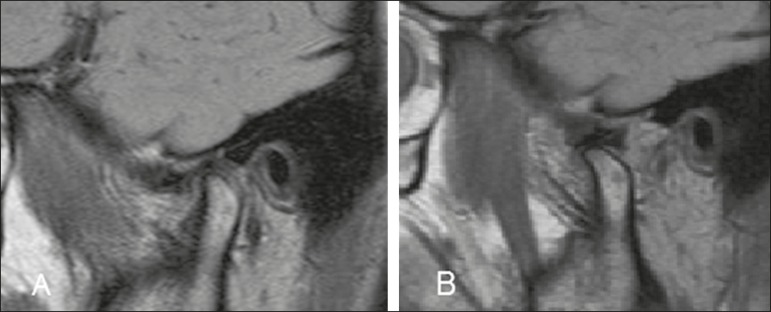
MRI showing DDWR. A: Closed-mouth position: articular disc
